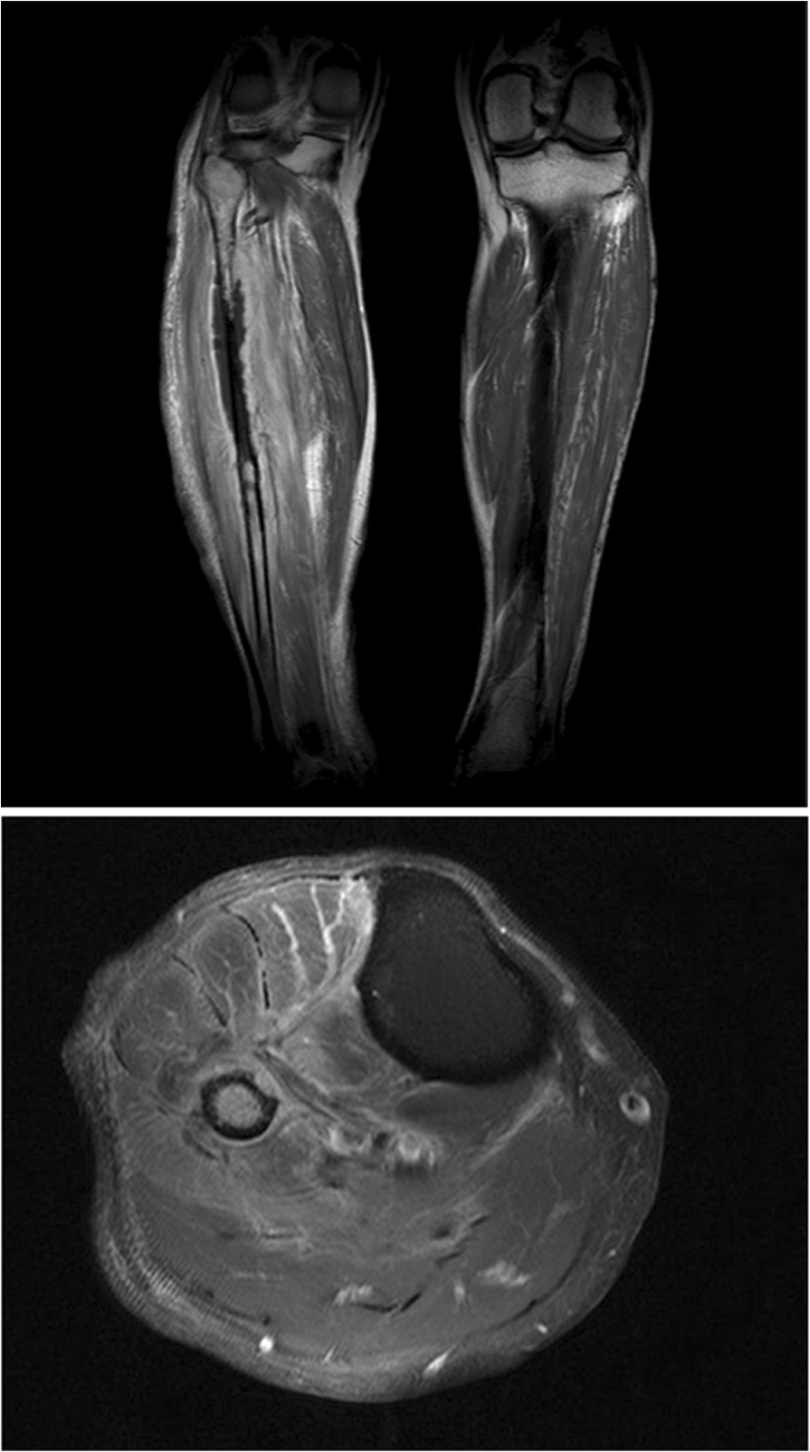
Enhanced magnetic resonance imaging of the right lower leg showing fibular enhancement and adjacent muscular abscess. (Fat saturated contrast enhanced T1W1).
